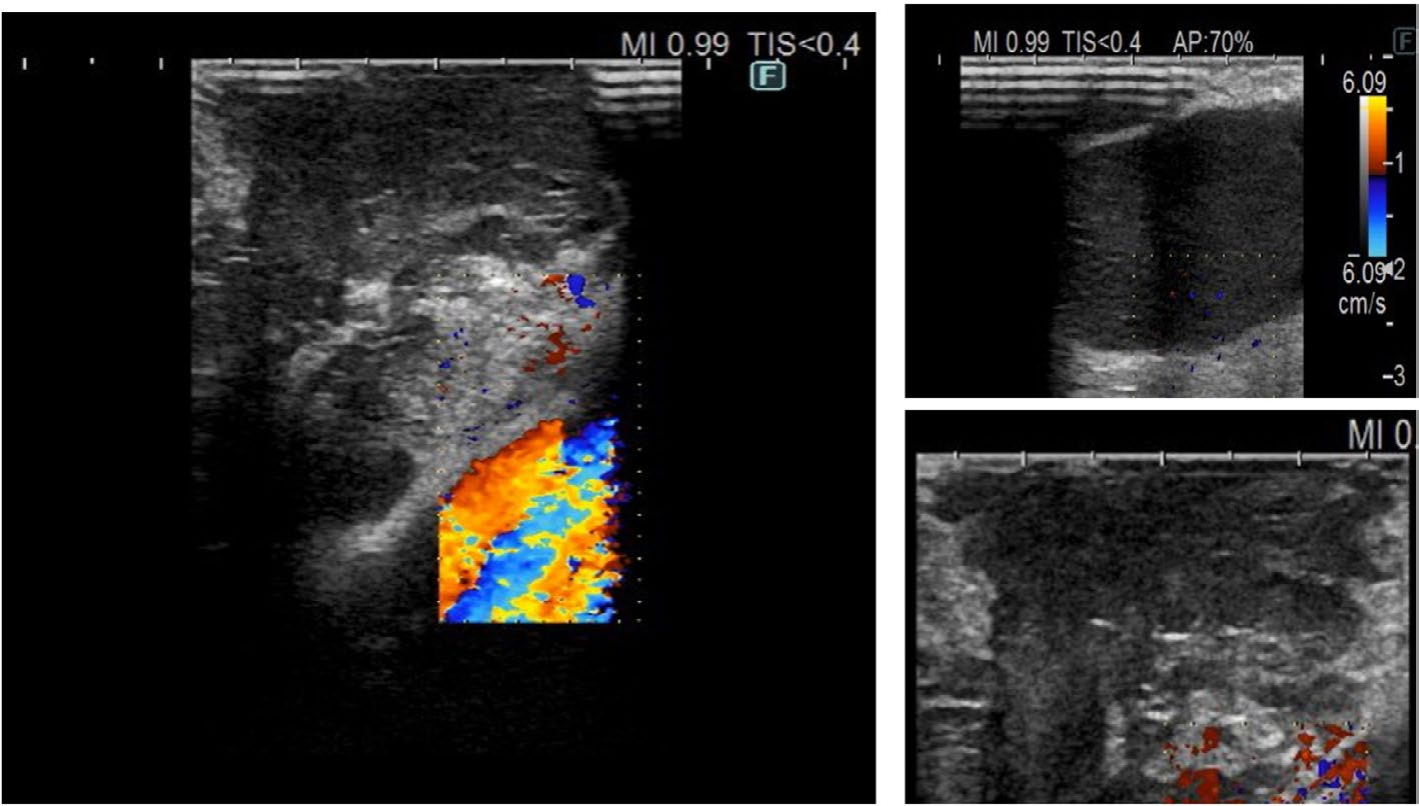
Using intraoperative ultrasound, we identified the exact site and solid part of the lesion where to perform the biopsies and indicated the depth and direction for the forceps.
